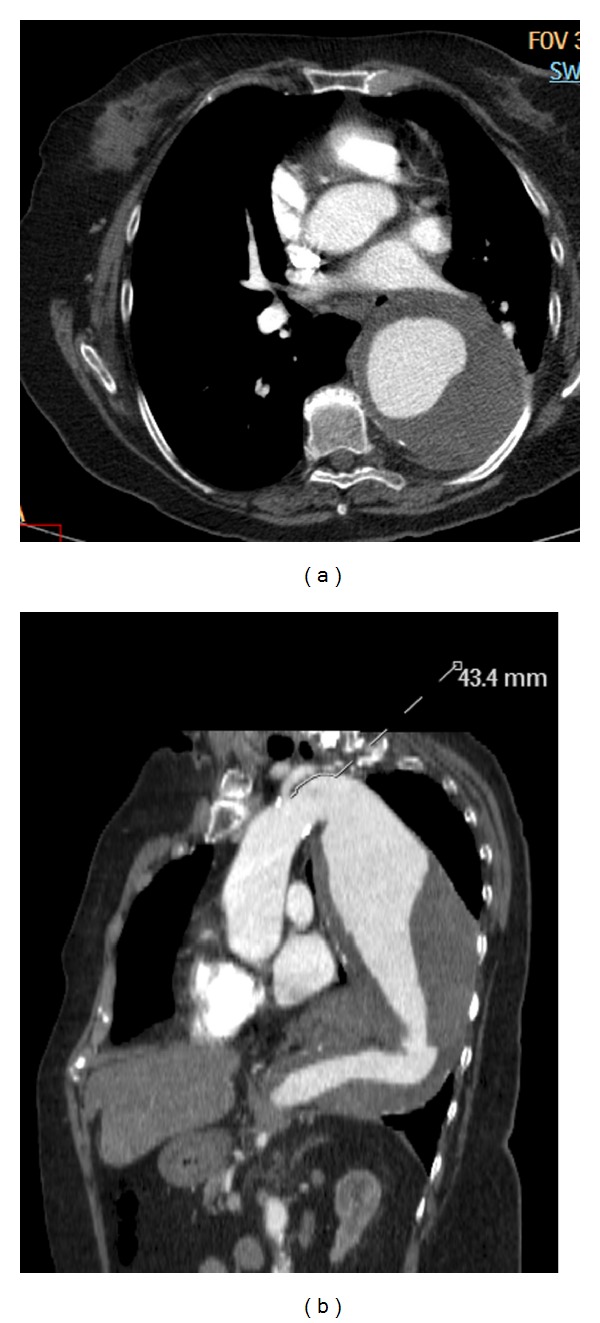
Descending  thoracic  aortic  aneurysm—(a) axial CT scan image shows a large aneurysm of the descending thoracic aorta, with large intraluminal thrombus. (b) Oblique reconstructed CT image shows a large fusiform descending thoracic aortic aneurysm. There is a 4.3 cm distance between the neck of the fusiform aneurysm and the left subclavian artery, which makes this patient suitable for endovascular repair.
